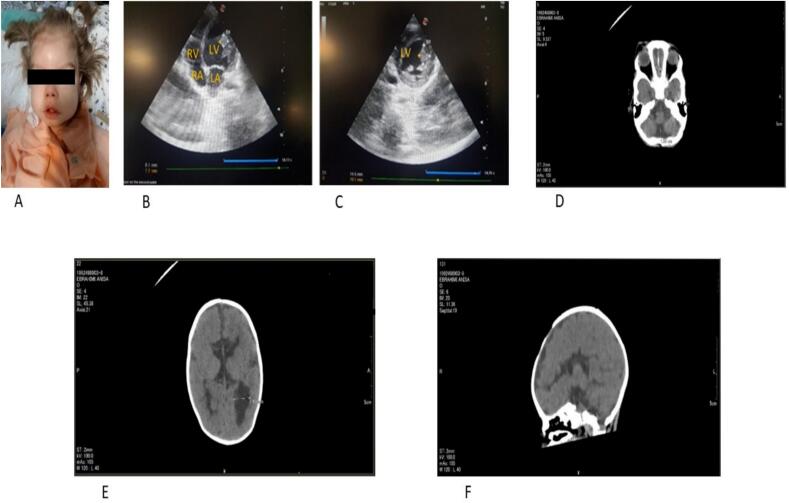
(B and C): Transthoracic echocardiogram in 4 chamber (B) and short axis view (C) RV: right ventricular, LV: left ventricular, LA: left atrium, RA: right atrium. D: Axial view, Focal enlargement of the CSF-filled subarachnoid space is seen in the posteroinferior portion of the posterior cranial fossa, consistent with enlarged cisterna magna maybe due to hypoplasia or agenesis of the vermis. E: axial view. F: sagittal view. E, F: The anterior horns of lateral ventricles are small, with parallel widely separated bodies - the “racing car” sign, with the third ventricle sited highly. There is dilation of the occipital horns of the lateral ventricles, corresponding to colpocephaly. The corpus callosum and septum pellucidum are absent.
